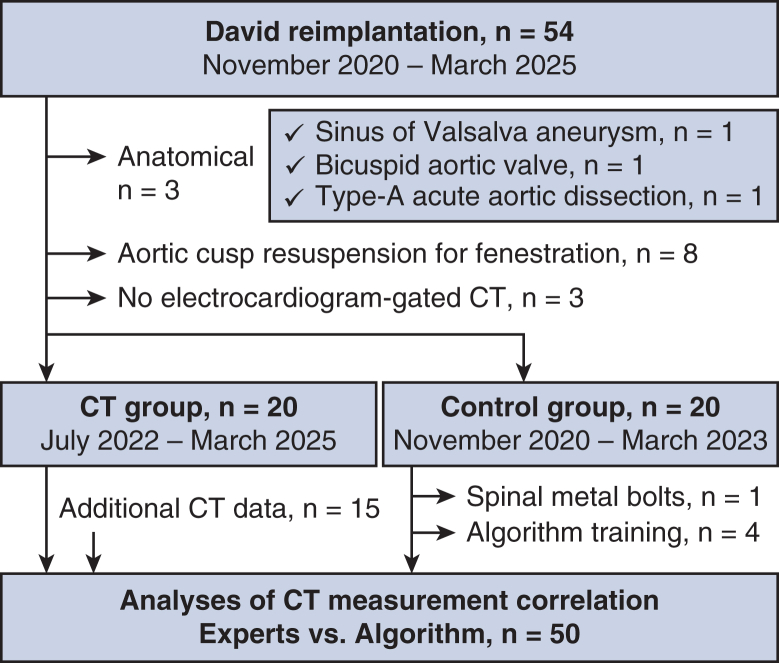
Study eligibility criteria. *CT*, Computed tomography.
